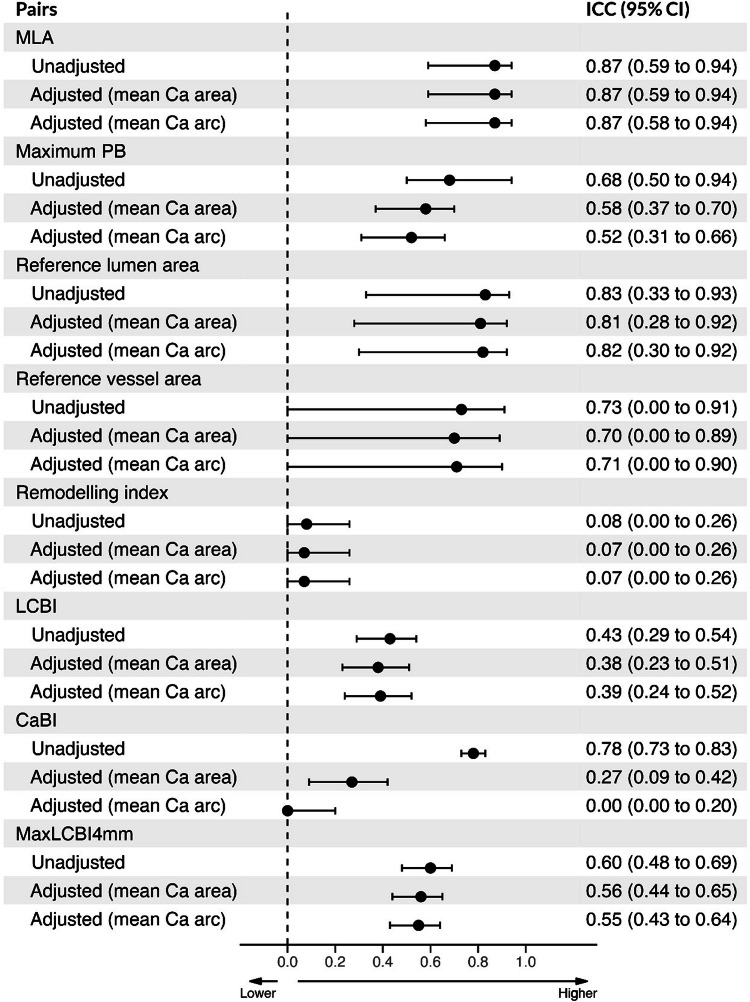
Unadjusted and adjusted (by mean Ca area and mean ca arc) ICC and 95% CI for measurements between NIRS-IVUS and CTA estimated by linear mixed model at lesion level
